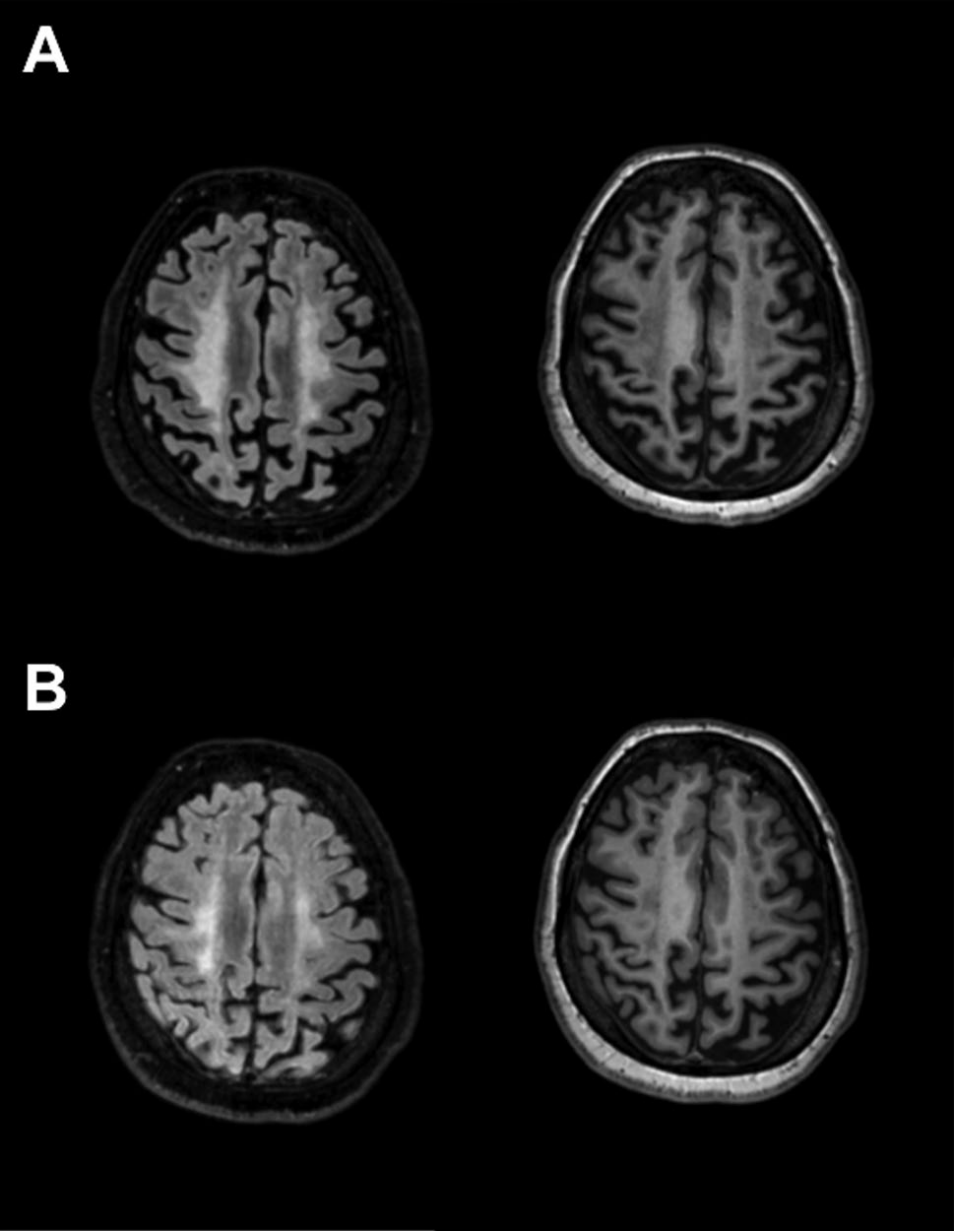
Baseline and follow-up MRI after 3 month of the index patient. Axial T2-weighted FLAIR (left) and corresponding T1-weighted (right) image of the index-patient at baseline (A) and follow-up (B) investigation showing substantial regression of the hyperintense (T2), respectively, hypointense (T1) areas, reflecting lesion volume reduction, which could be objectified by LPA-based lesion quantification (baseline 62.11 ml and follow-up 21.69 ml T2 lesion volume)
